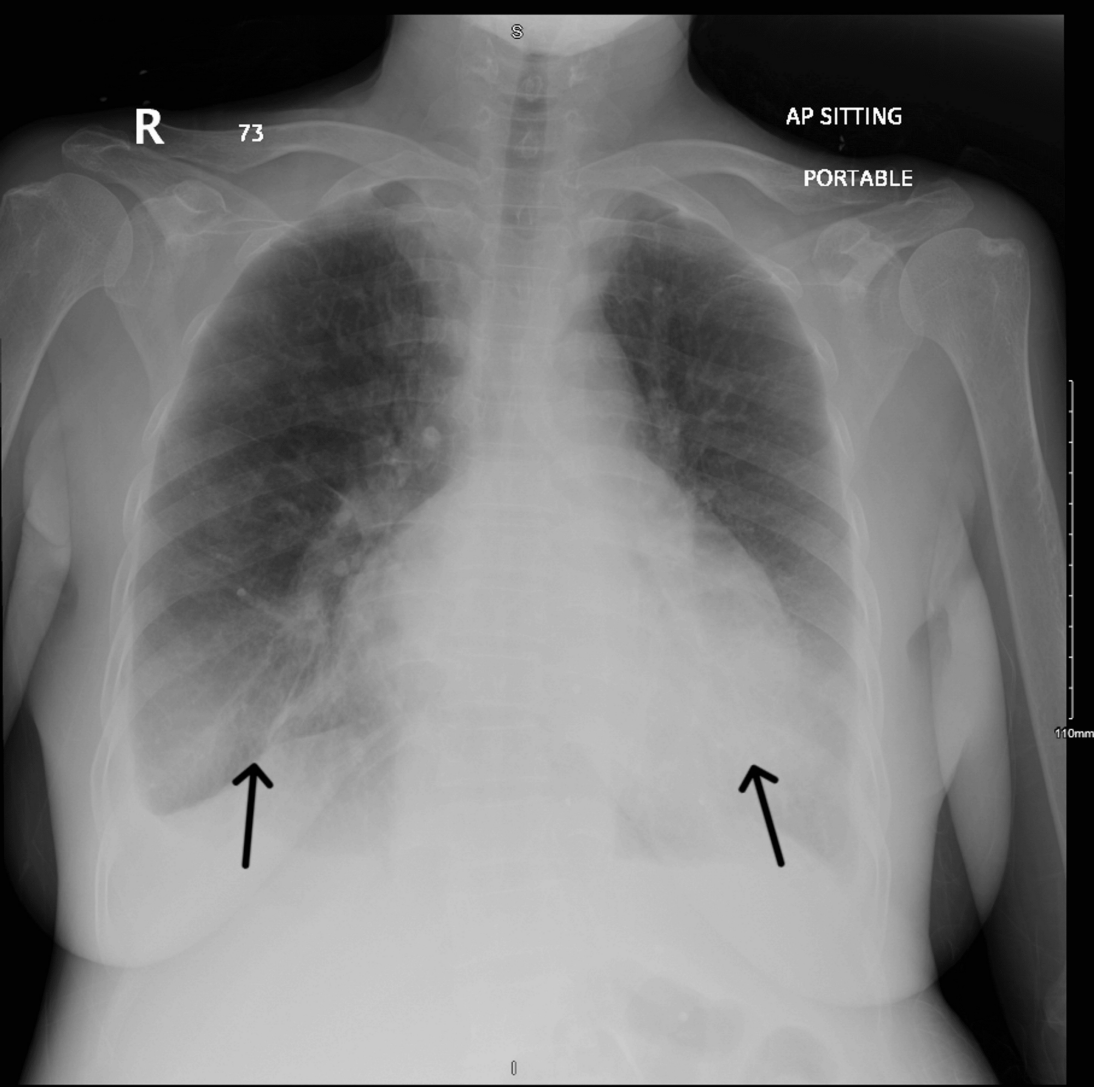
Chest X-ray showing pleural effusion (black arrows).
